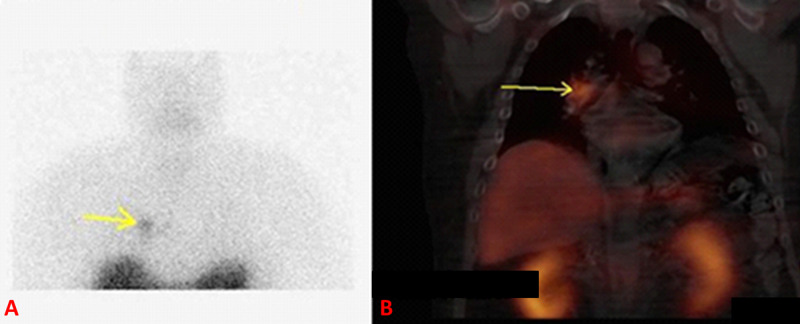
(A) Planar imaging of octreotide scan showing the right hilar carcinoid tumor (arrow), (B) single photon emission computed tomography of same lesion (arrow).
